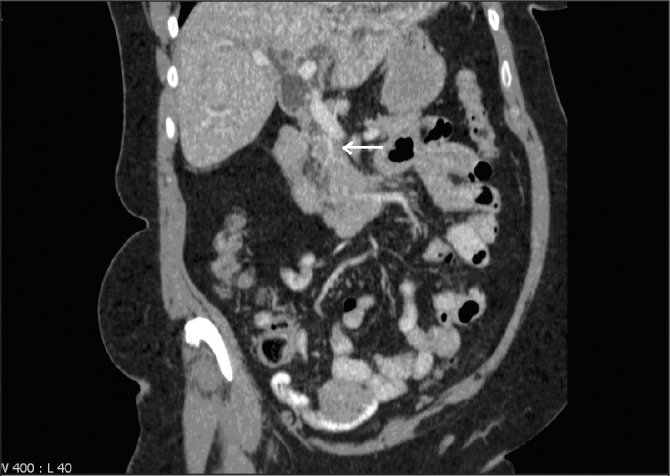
Coronal section of contrast-enhanced computed tomography of the abdomen and pelvis demonstrating a stricture at the mid-common bile duct (arrow)
